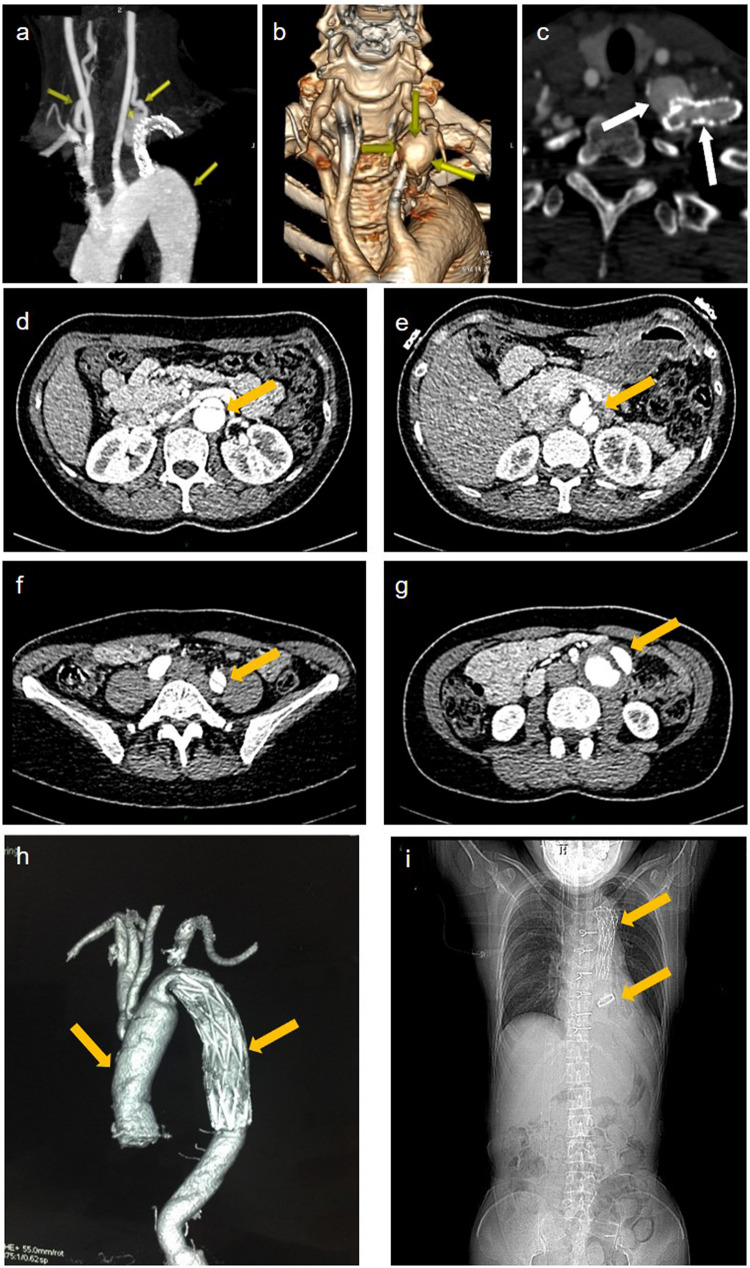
Imaging data of the patient. (a) representative maximum intensity projection picture of the left subclavian artery which has been stented to exclude the aneurysm. (b) volume rendered (vr) three-dimensional (3d) reconstructed images show a large aneurysm of the left subclavian artery near its origin from the aortic arch. (c) the cross section showed an opacification of the aneurysm with contrast agent seen with the parent vessel indicating an endoleak. (d–f) aortic ct angiography found debakey type iii dissecting aortic aneurysm ranged from the opening of the celiac trunk (e), the proximal rupture located at the level of the bilateral renal artery (d), and ended at the left internal iliac artery (f). (g) the false lumen of the lower abdominal aorta showed aneurysmal dilatation and mural thrombosis. (h) the endovascular stent–graft placement was shown. (i) x-ray showing thoracic incision and metal valves after aortic valve replacement.
